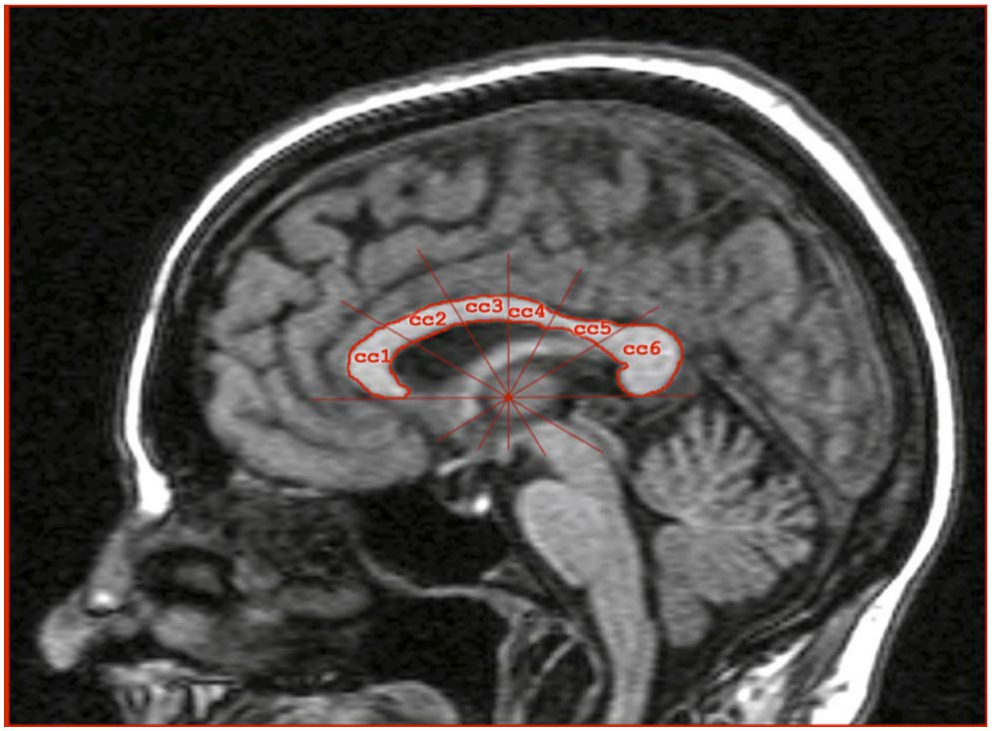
T1-weighted mid-sagittal view of the corpus callosum (CC). Six sub regions are shown: CC1 (genu), CC2, CC3, CC4, CC5 and CC6 (splenium).
